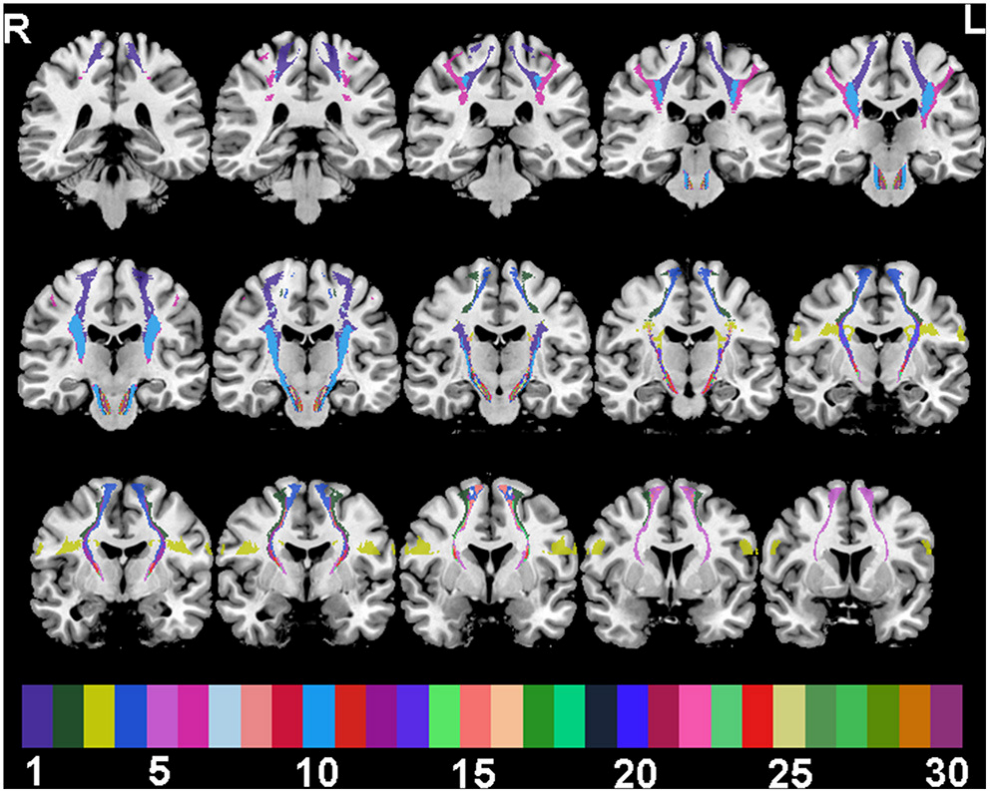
SMATT tracts in a random colour-scale superimposed on 15 grayscale coronal T1-weighted anatomical slices. Colour indices are: 1 M1; 2 PMd; 3 PMv; 4 SMA; 5 preSMA; 6 S1; 7 M1,PMd; 8 M1,PMv; 9 M1,SMA; 10 M1,S1; 11 PMd,PMv; 12 PMd,SMA; 13 PMv,SMA; 14 PMd,preSMA; 15 SMA,preSMA; 16 M1,PMd,SMA; 17 M1,PMv,SMA; 18 M1,PMv,S1; 19 M1,SMA,S1; 20 PMd,PMv,SMA; 21 M1,PMd,SMA,S1; 22 M1,PMv,SMA,S1; 23 M1,PMd,PMv,SMA; 24 PMd,SMA,preSMA; 25 M1,PMd,PMv,SMA,S1; 26 M1,PMd,SMA,preSMA; 27 PMd,PMv,SMA,preSMA; 28 M1,PMd,SMA,preSMA,S1; 29 M1,PMd,PMv,SMA,preSMA; 30 M1,PMd,PMv,SMA,preSMA,S1.
